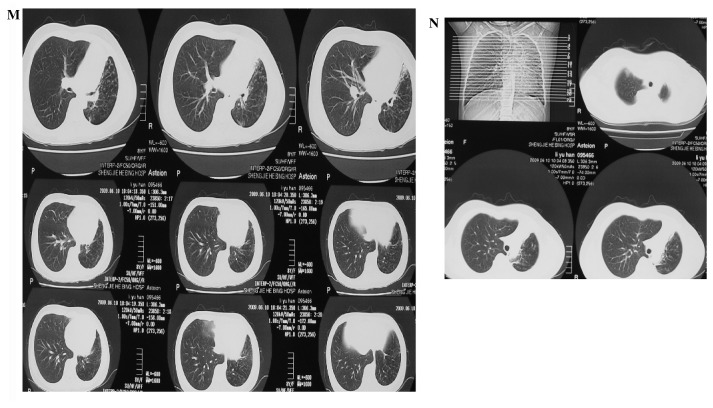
(A) Computed tomography (CT) imaging 2 weeks before treatment (03/01/2009); (B) CT imaging following anti-pulmonary tuberculosis (PTB) therapy (03/19/2009); (C) left main bronchus; (D) argon plasma coagulation; (E) insertion of guide wire; (F) prior to first balloon dilation; (G) following first balloon dilation; (H) rechecked bronchus partial coarctation; (I) second balloon dilation; (J) fourth rechecking; (K) third balloon dilation. (L) Left main bronchus was unobstructed with partial bronchostenosis. (M and N) computed tomography (CT) imaging following treatment (06/10/2009).
